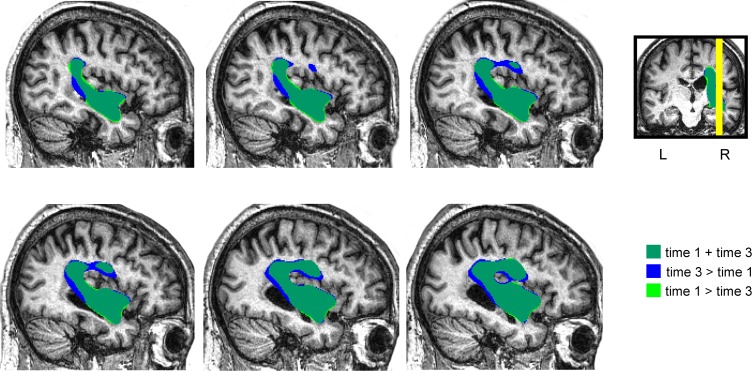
Depiction of the patient’s lesion mask in the time point 1 (BL) and in the time point 3 (DP + T) over the patient’s normalized sagittal T_1_-weighted image. The lesion mask drawn of the initial MRI (16 months post-stroke onset) was larger in the third MRI (28 weeks after study entry) due to expansion of its subinsular component. The observed lesion expansion probably resulted from retraction of cortical tissues due to focal temporal-parietal post-stroke atrophy (see Figure 2).
